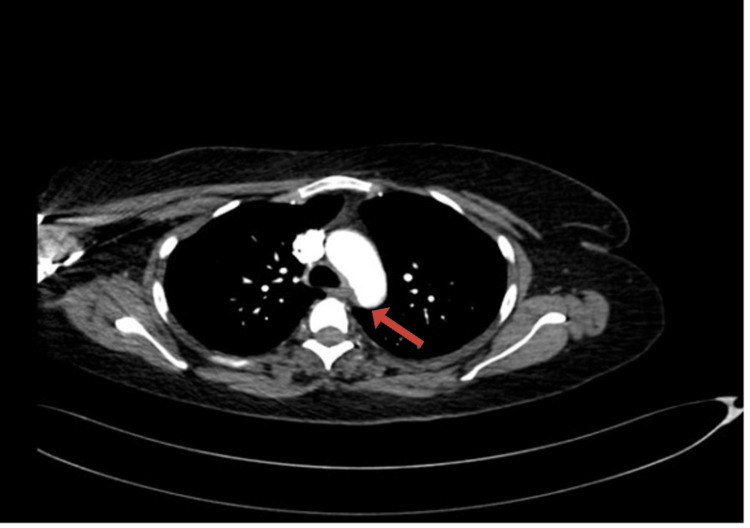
Axial CT chest after 42 days of anticoagulation showing a reduction in the size of the eccentric mural thrombus.
